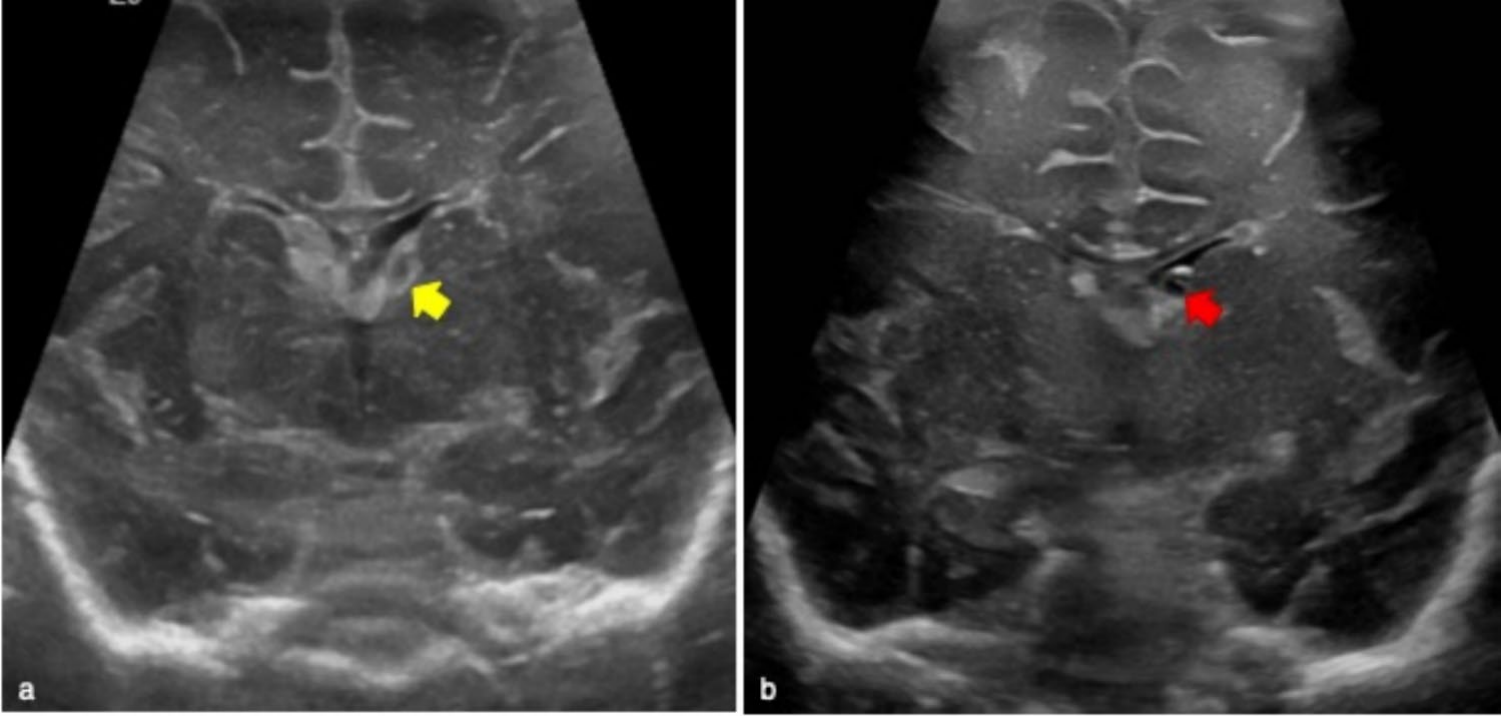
Follow-up ultrasound of premature male infant born at 34 weeks gestation demonstrated progressive evolution of grade 2 left subependymal germinal matrix hemorrhage. In a intermediate phase of colliquation (yellow arrow in a), followed by subependymal cyst evolution after 2 weeks (red arrow in b)
